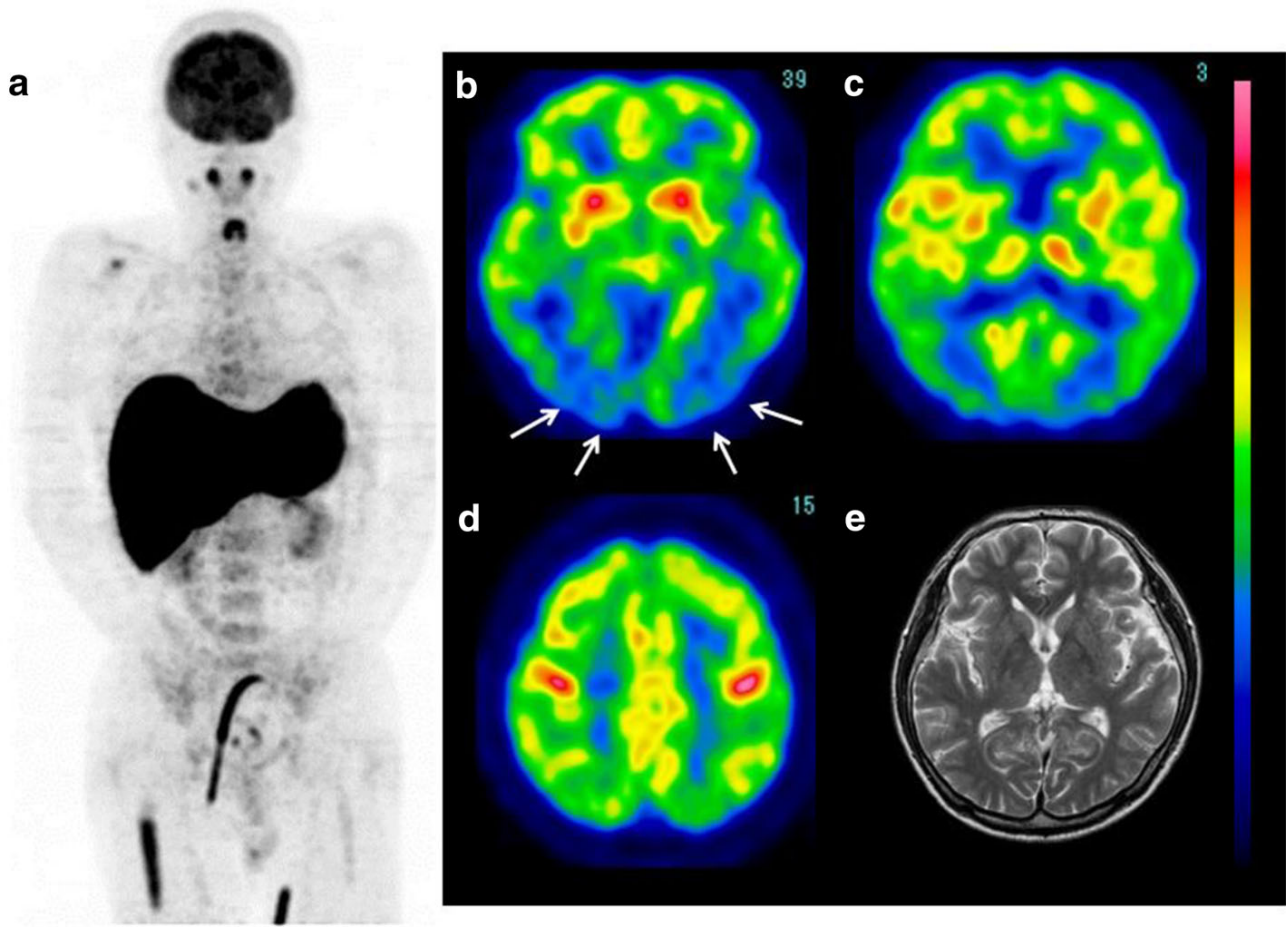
(Case 1: diffuse large B-cell lymphoma, intravascular lymphoma) FDG-PET images of the whole body and brain. Whole-body FDG-PET showed a high uptake in the liver and bilateral femoral bone marrow (a). Brain FDG images demonstrated a reduction in glucose metabolism in the bilateral parieto-occipital regions (b–d). MRI showed no abnormalities in the brain (e)
